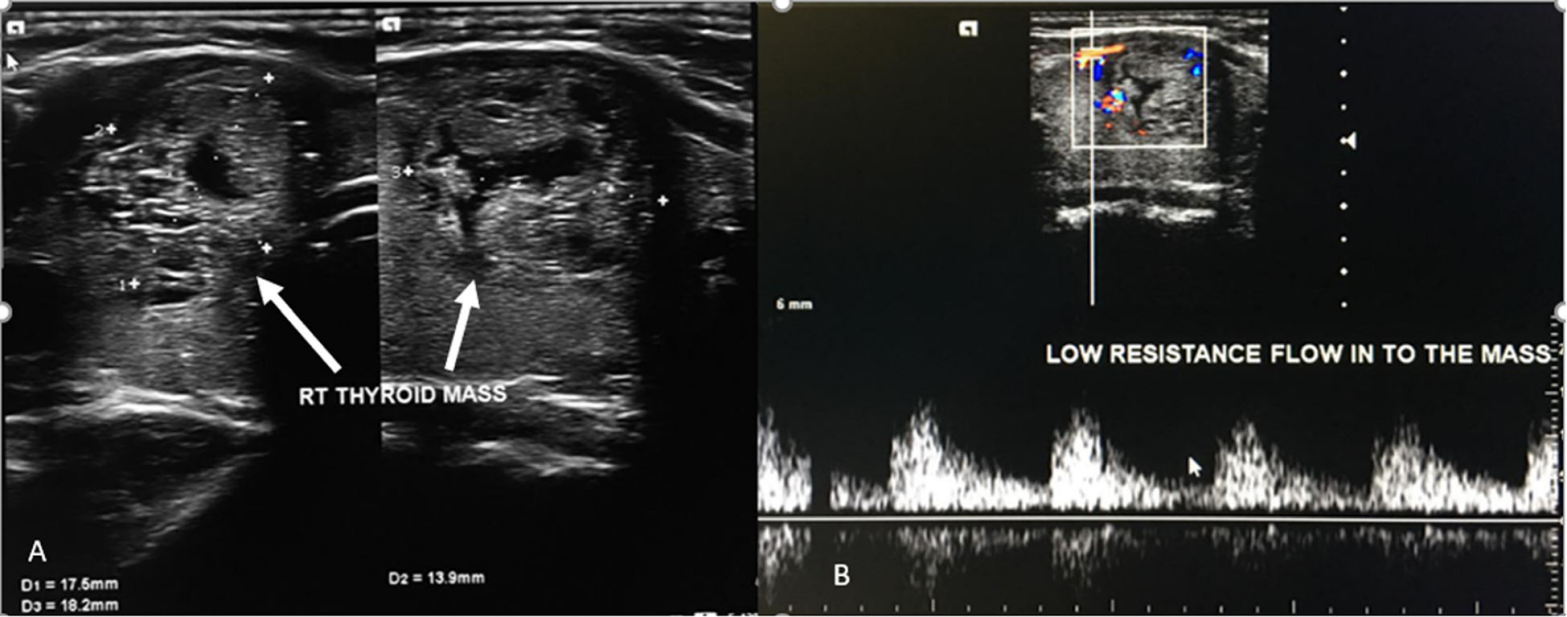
Ultrasound of the right thyroid nodule a a well-defined nodule, with smooth margins, wider than taller, hypoechoic, spongiform composition, and with no echogenic foci b Color and spectral Doppler (triplex Doppler) of the nodule showing moderate and low resistance flow respectively
